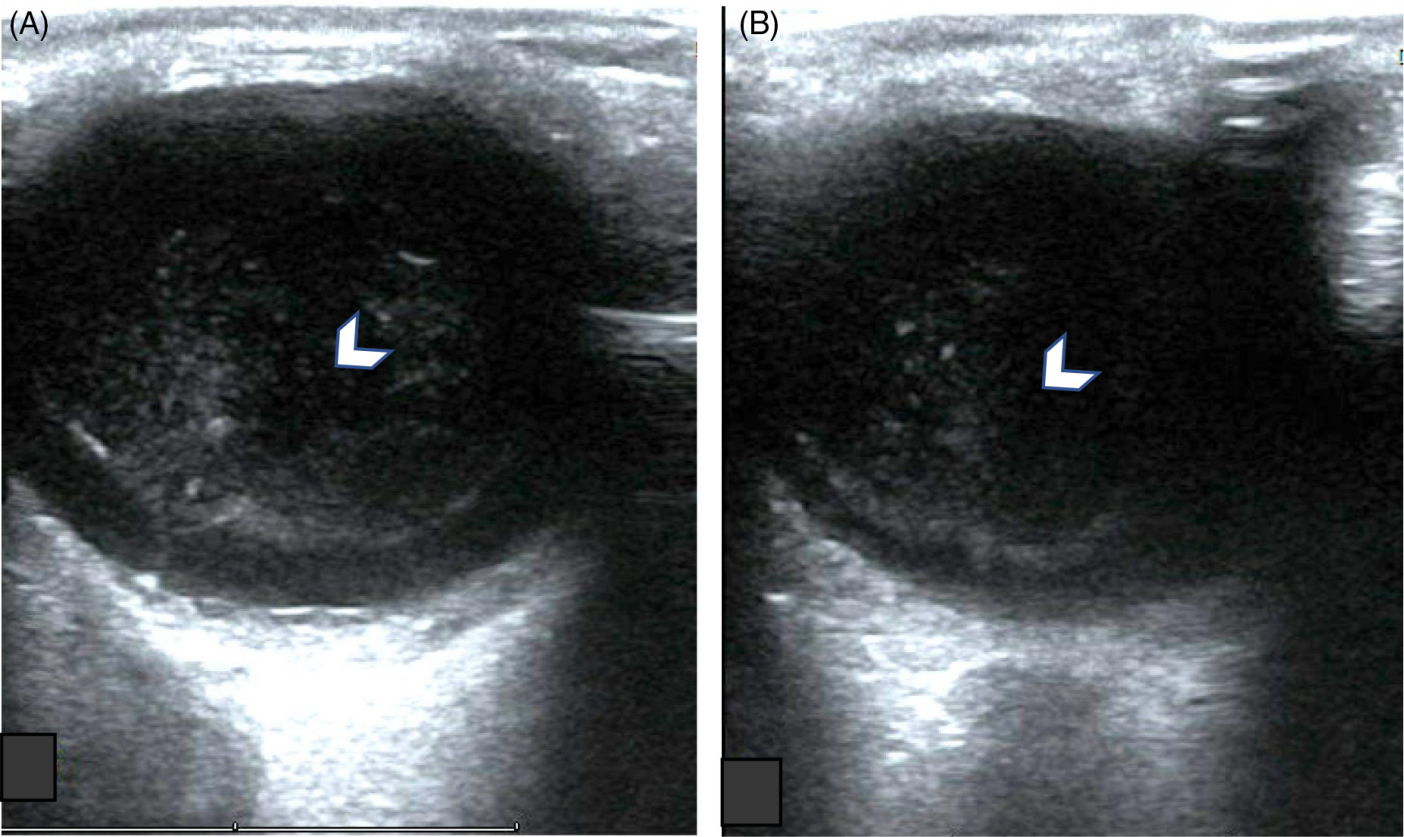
Ocular point-of-care ultrasound using the linear probe. (A) Transverse view of the orbit. (B) Sagittal view of the orbit. Subtle echogenic material is seen floating in the vitreous (arrowheads).
